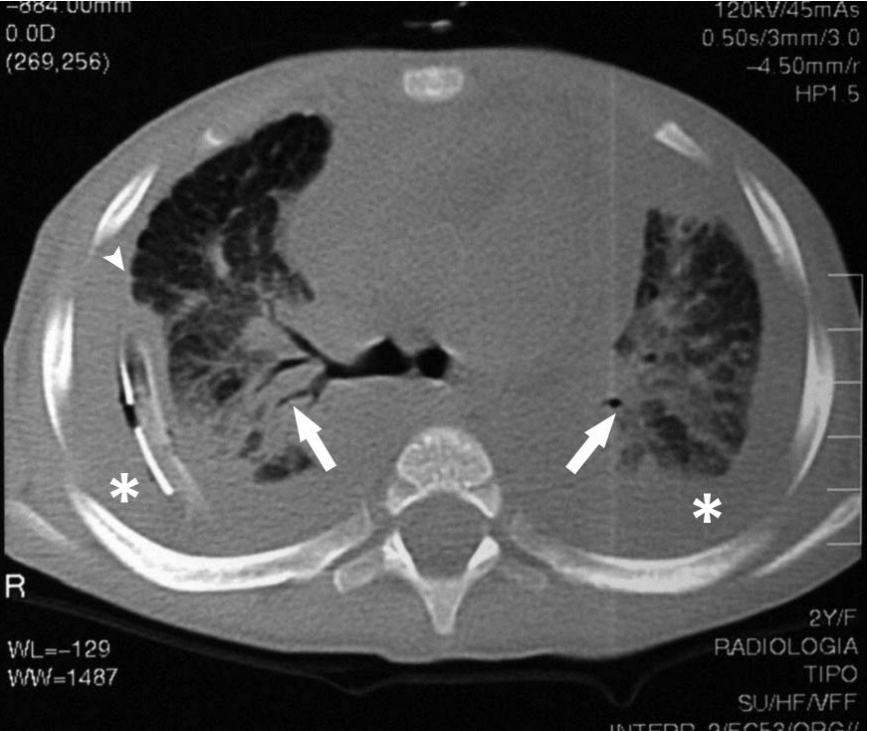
Pulmonary Lymphangiectasia. High-resolution computed tomography (HRCT). Diffuse thickening of the peribronchovascular interstitium and the interlobular septa (arrowheads), associated with bilateral pleural effusion (*), and peribronchovascular infiltrates (arrows) with bronchogram.
